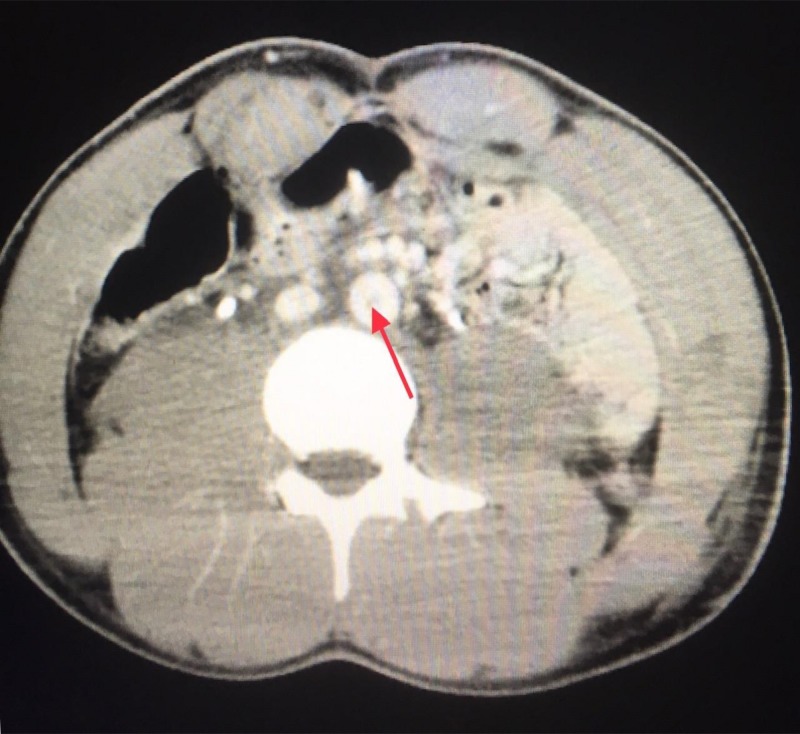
Computed tomographic scan of the abdomen and pelvis. Filling defect in the aorta (Red arrow) indeterminant for clot or mixing of contrast defects
